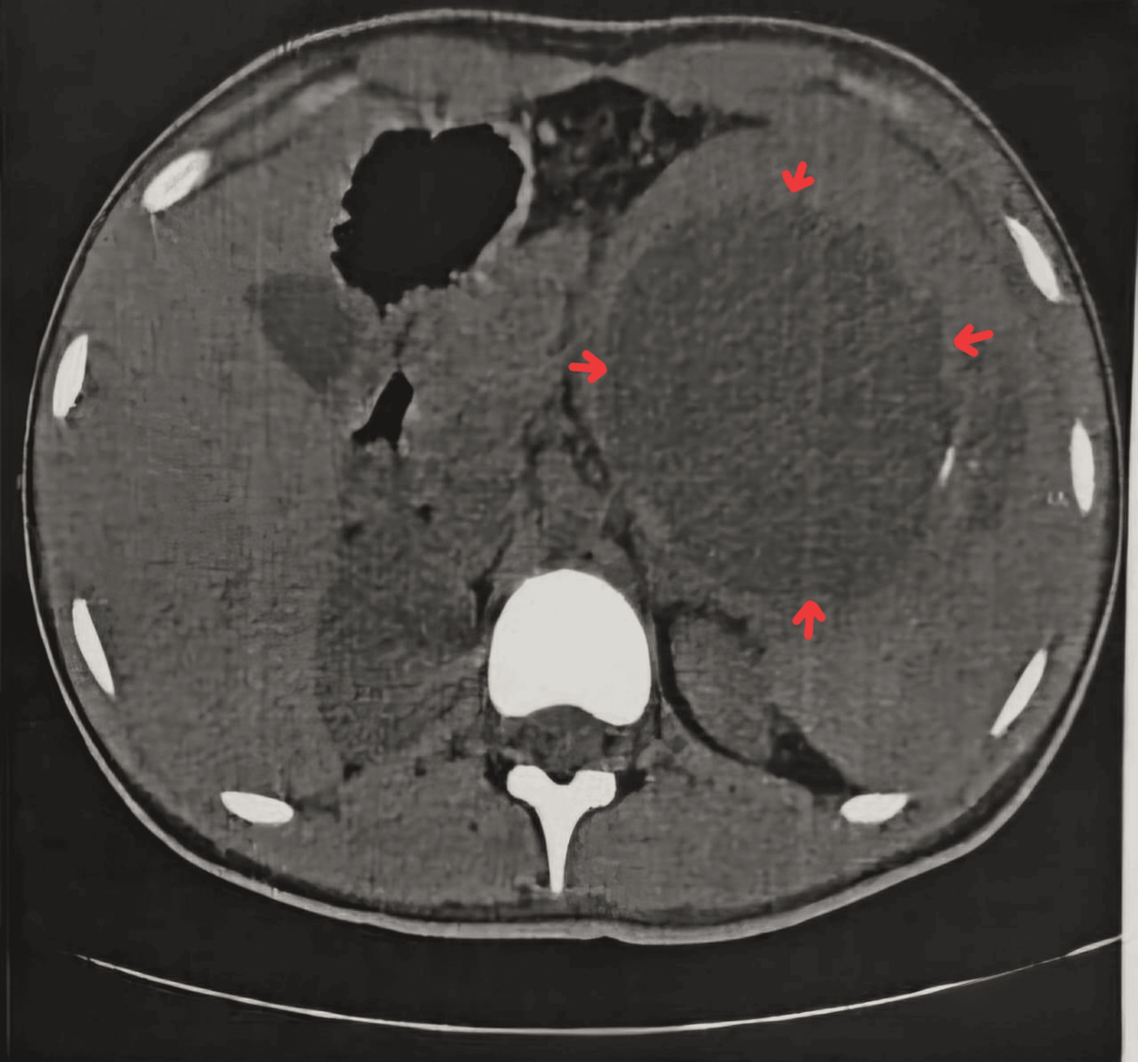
CECT of the abdomen (axial view) This image shows gross splenomegaly with a large cystic lesion (red arrows) measuring approximately 1500 cc, with dense internal contents and a few lobulations within the splenic parenchyma at the upper and mid-pole, extending up to the subcapsular location. There is no solid component or enhancement. The cyst is seen abutting the inferior surface of the left lobe of the liver and the tail of the pancreas, with the stomach displaced to the right side, and no encasement of the splenic vessels. CECT, Contrast-Enhanced Computed Tomography
